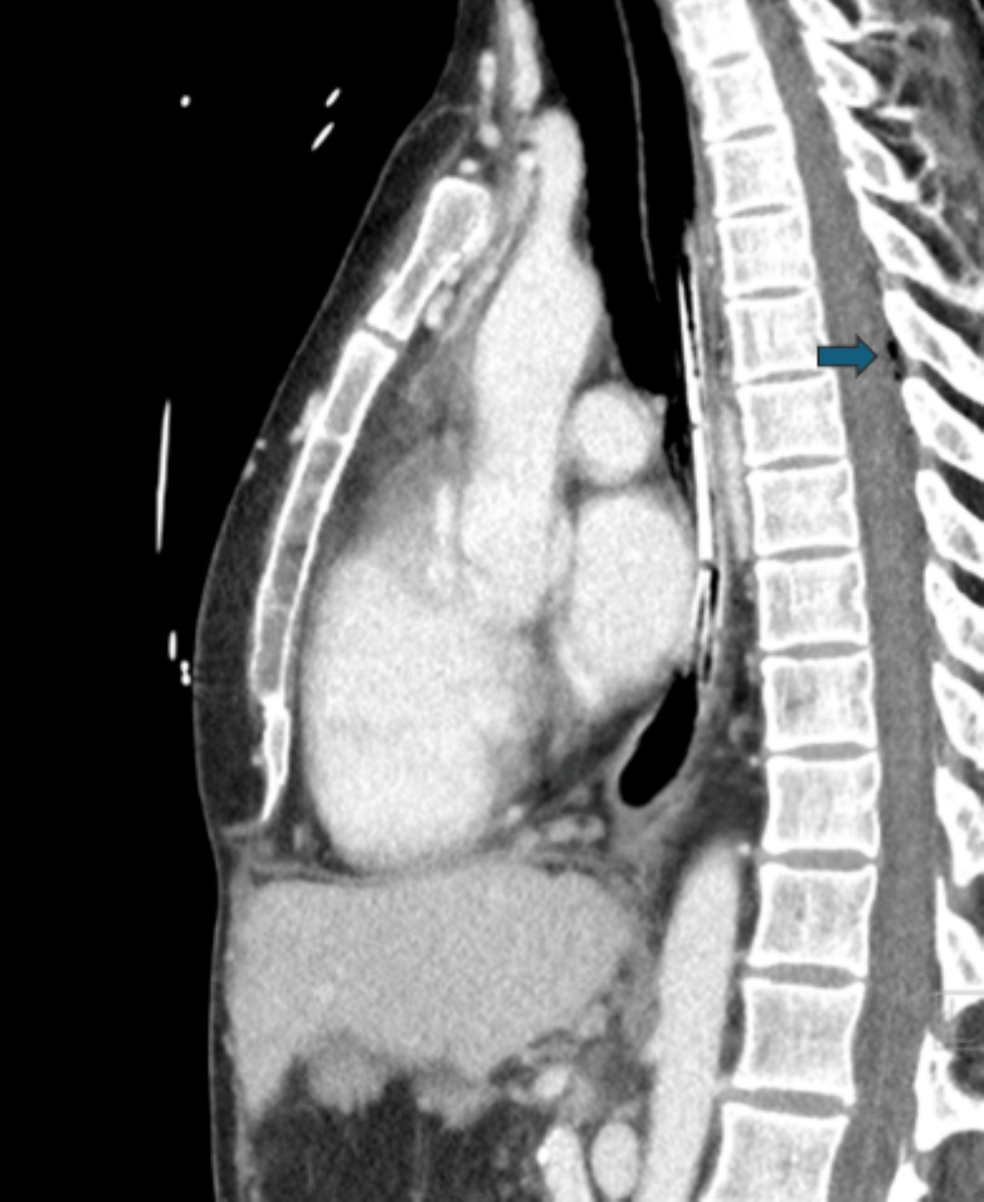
Computed tomography of the thoracic spine without contrast demonstrating epidural infusion at T7-T8
